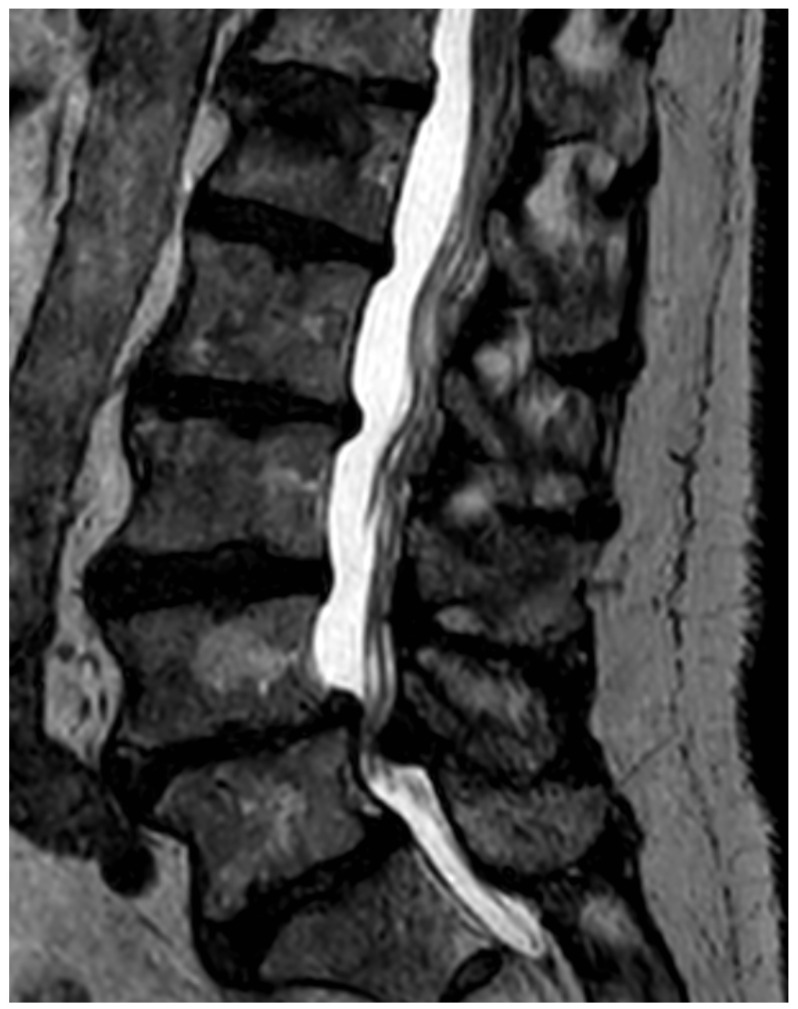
Typical sagittal T2 sequence of a lumbar MRI with signal-attenuated and reduced-height intervertebral discs, evidence of multisegmental disc bulging and degenerative spinal stenosis in segment L4/L5. Additionally, typical vertebral haemangioma at L4.
